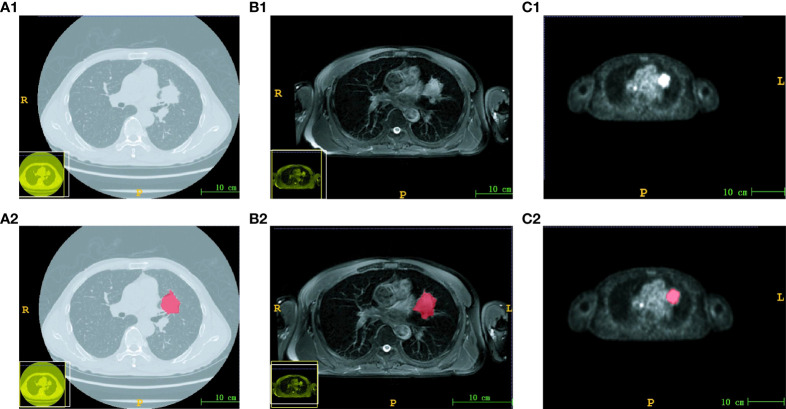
Images and corresponding ROI of three modalities. The original image of CT (A1), MRI (B1) and PET (C1). The ROI image of CT (A2), MRI (B2) and PET (C2). The scale bar was 10 cm.
